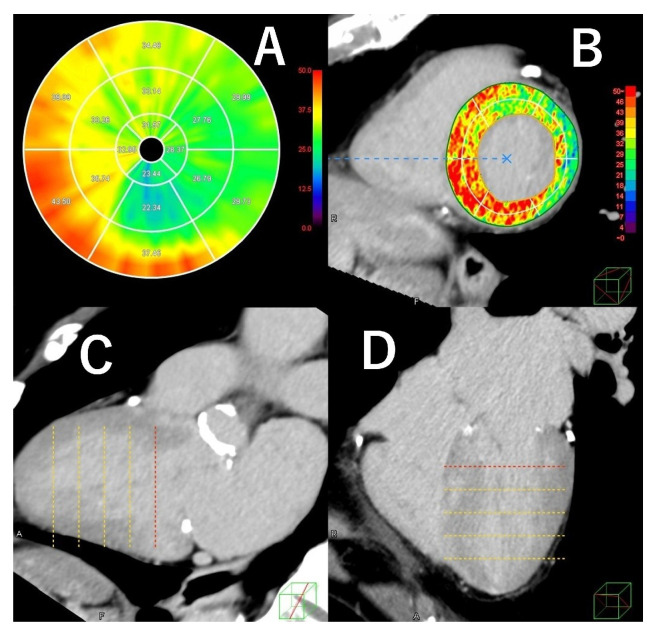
This is a case of severe aortic stenosis in which ECV analysis of the left ventricular myocardium (LVM) was performed using pre-TAVI CT (A). The mean ECV of the LVM was 33.6%. This patient was diagnosed with cardiac amyloidosis two years after TAVI. The short-axis view, two-chamber view, and four-chamber view of delayed contrast LVM are shown in Figures (B–D).
